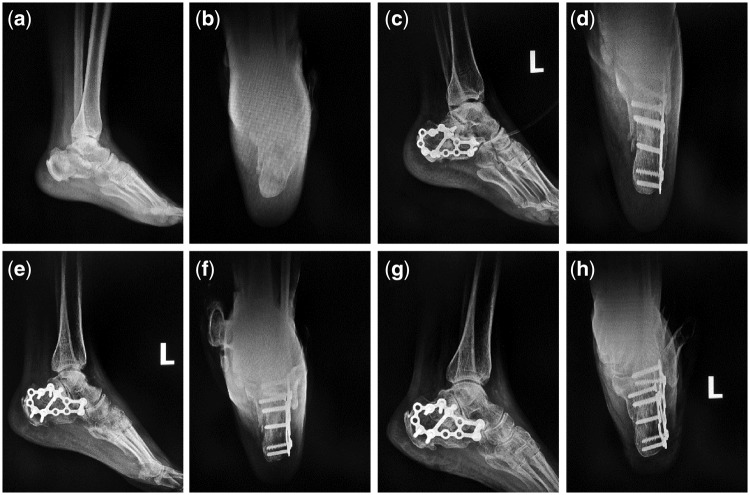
Plain radiographs of the second typical case. (a and b) Preoperative lateral and anteroposterior films; (c and d) immediately postoperative lateral and anteroposterior films; (e and f) 3 months postoperative lateral and anteroposterior films; (g and h) 12 months postoperative lateral and anteroposterior films)
